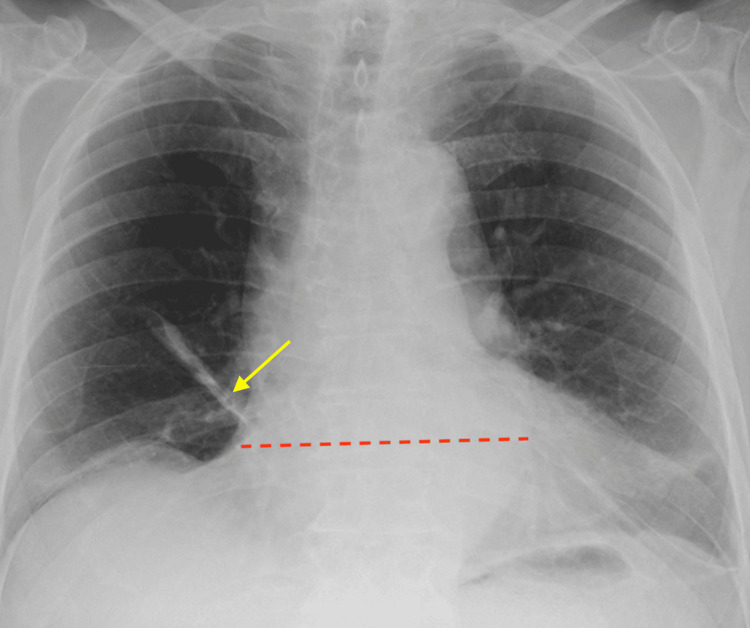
Bibasal linear atelectasis chest X-ray of the patient Yellow arrow denote linear atelectasis; the dotted red line measures the enlarged cardiac silhouette (cardiomegaly).
